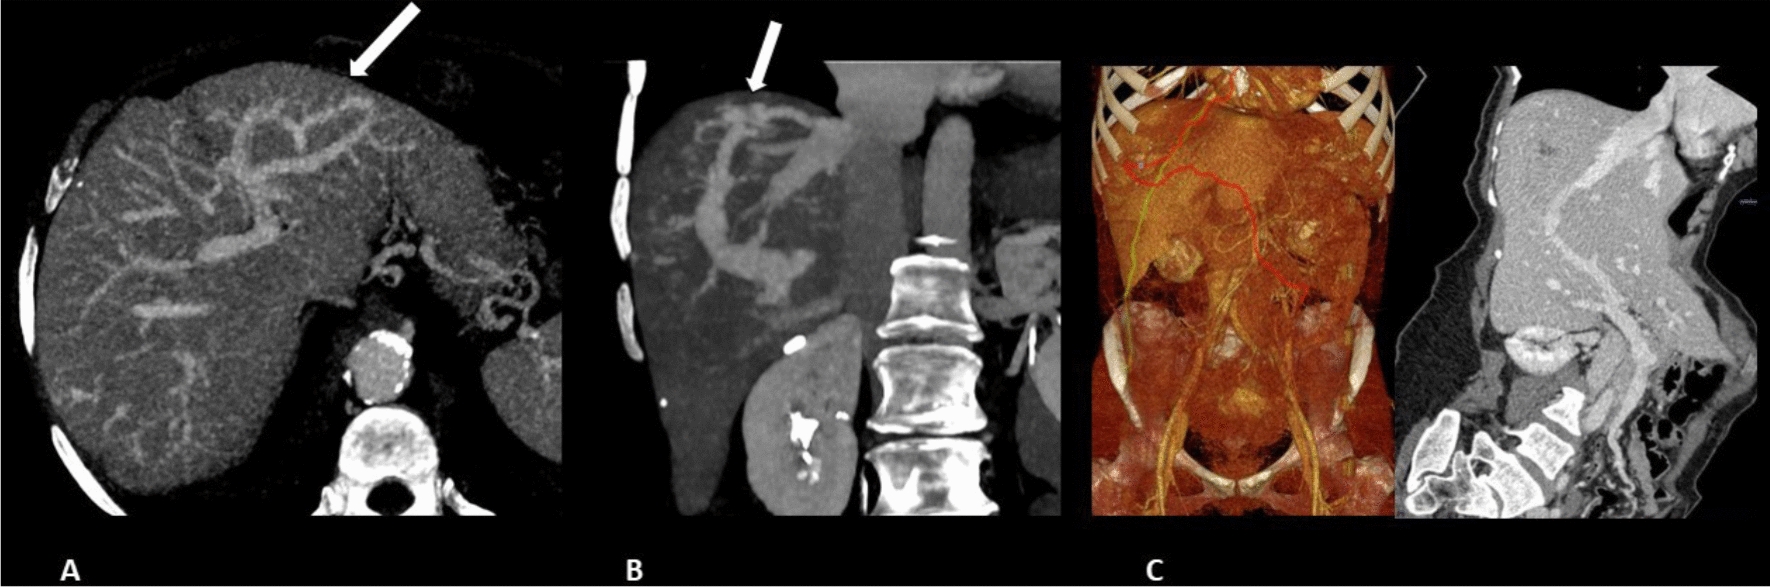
A Axial MIP (maximum intensity projection) computed tomography (CT) image obtained in the hepatic phase showing portohepatic venous shunting in the left hepatic lobe. B Coronal MIP CT image showing portohepatic venous shunting, with dilated portal vein communicating the right hepatic vein through a focal vascular mass. C 3D CT reconstruction showing portovenous shunting with the right hepatic vein
